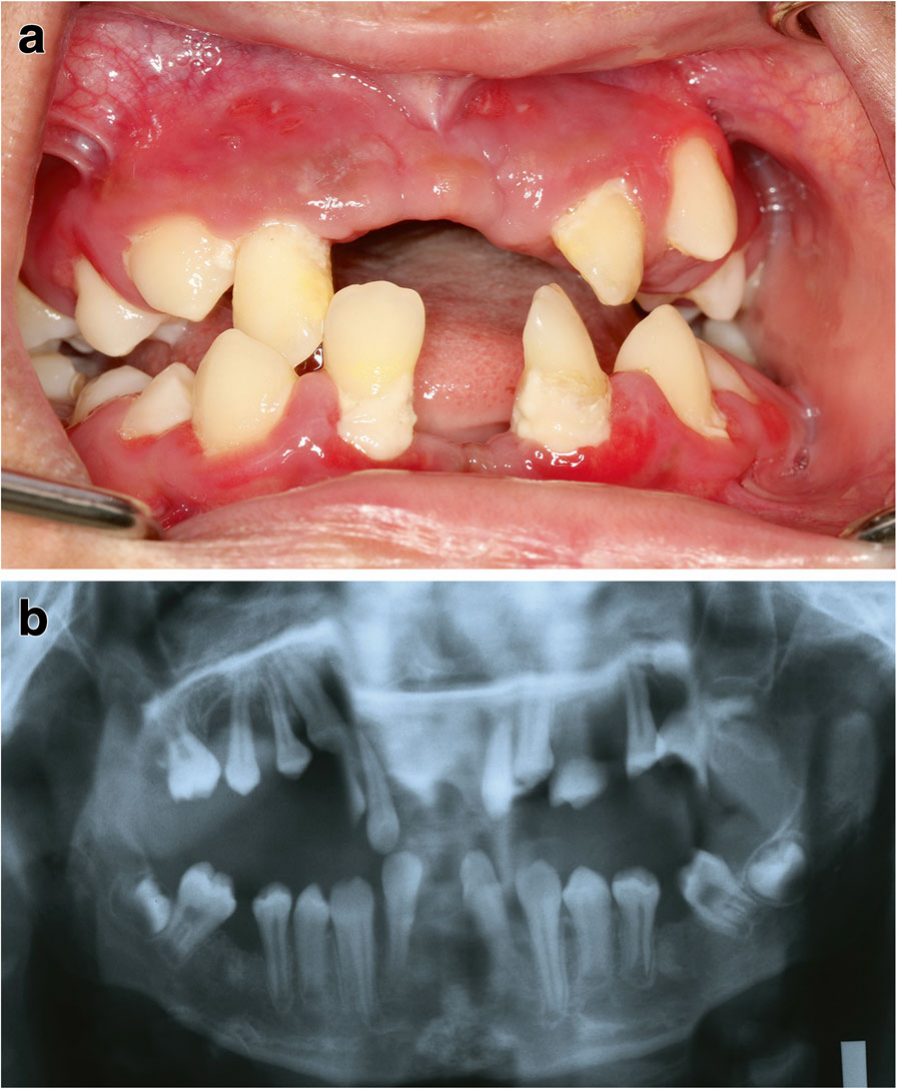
Case 5. a Intraoral appearance with loss of permanent anterior from both jaws, severe inflammation, and enlargement of the gingiva. b Panoramic radiograph showing severe destruction of the alveolar bone and loss of permanent anterior teeth
